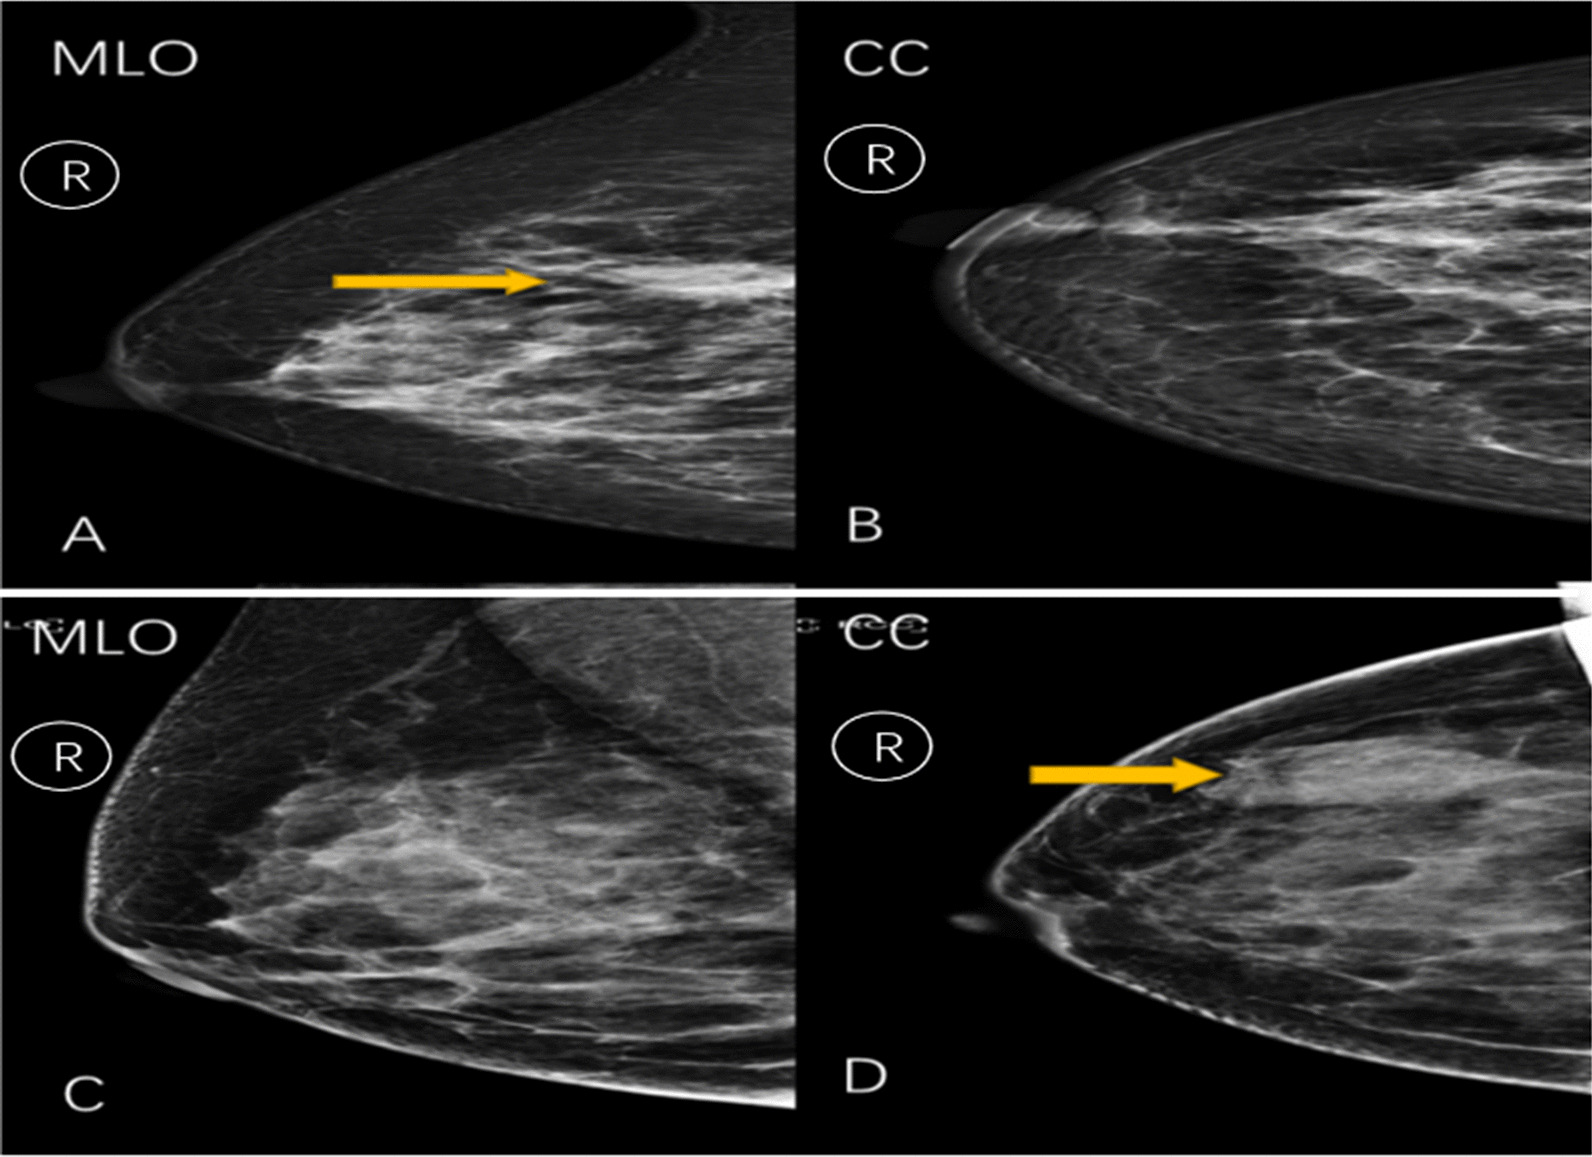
A and B The combination of surgical pathology, ultrasound, and MRI images of a patient (female, 48 years old) determines an irregular mass in the upper inner quadrant of the right breast. A A mass shadow over the right breast is seen in the MLO position in radiography. B No obvious lesion is seen in the CC position; Hence, we have chosen the radiomics features from the MLO. C and D The combination of surgical pathology, ultrasound, and MRI images of a patient (female, 65 years old) shows a well-defined mass in the upper outer quadrant of the right breast. C A mass shadow is seen in the MLO position in radiography. D No obvious lesion is seen in the CC position. C No obvious lesion is observed in the photographic MLO position. D A mass shadow is observed in the right lateral breast in the CC position. Hence, we have chosen the radiomics features from the CC
